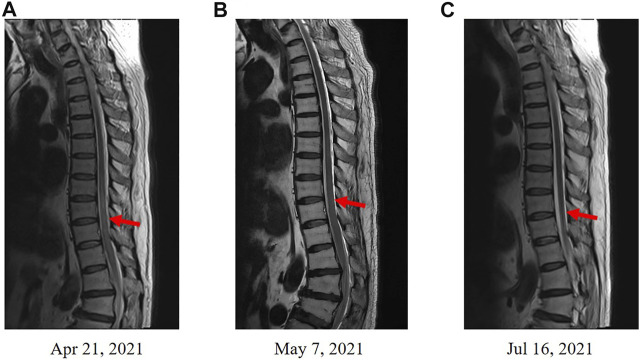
Enhanced MRI scans in spinal cord. (A) Enhanced MRI before the treatment of methylprednisolone. (B) Enhanced MRI revealed an improved condition after the treatment of methylprednisolone. (C) Enhanced MRI revealed a disappeared diffuse abnormal signal after 3 months.
